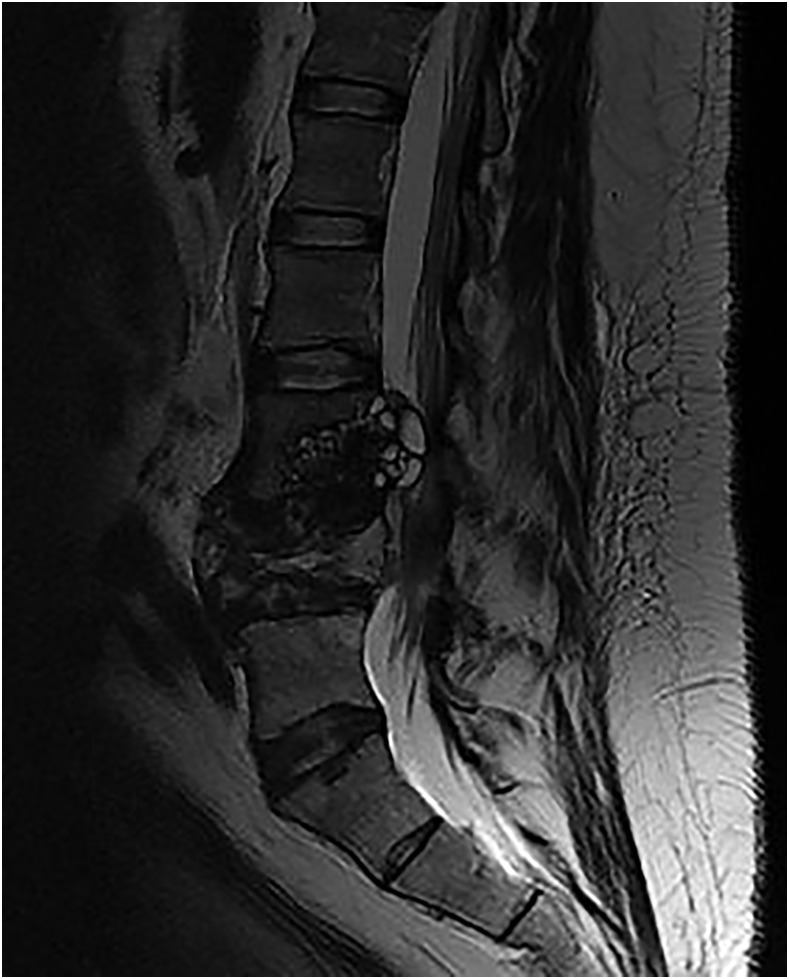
Sagittal magnetic resonance imaging (T2-weighted) of the lumbosacral spine showing an expansile multicystic lesion causing destruction of the fourth lumbar vertebra.
